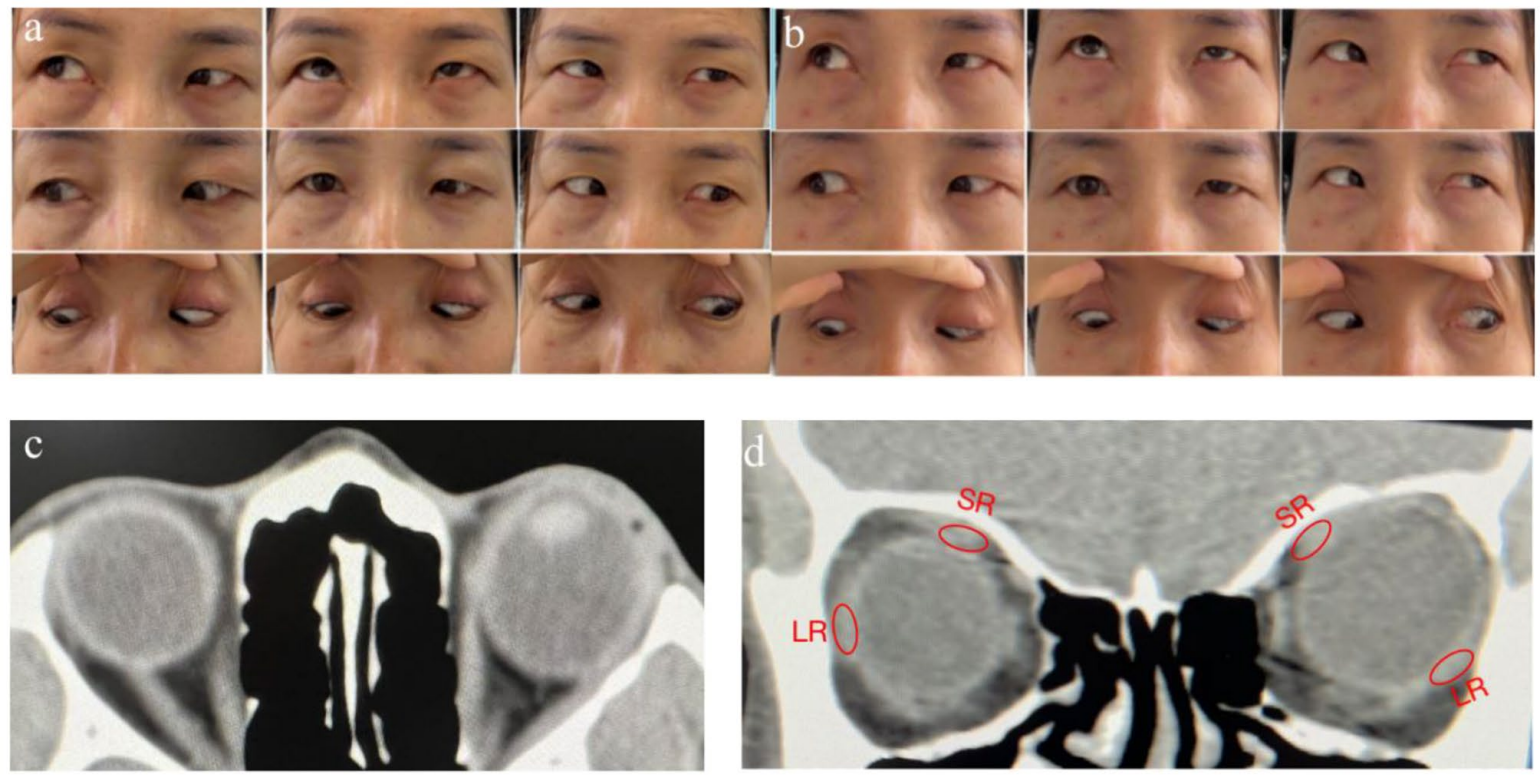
Patient’s photographs on a “bad” day and a “good” day, plus computed tomography (CT)scans. a “Bad” day.The patient manifested a 60 PD esotropia and 30 PD hypotropia in primary position with mild limitation of abduction in her left eye. b Orthotropia on “Good” day. c and d supertemporal dislocation of the posterior portion of the elongated globe out from the muscle cone in her left eye on CT scan
